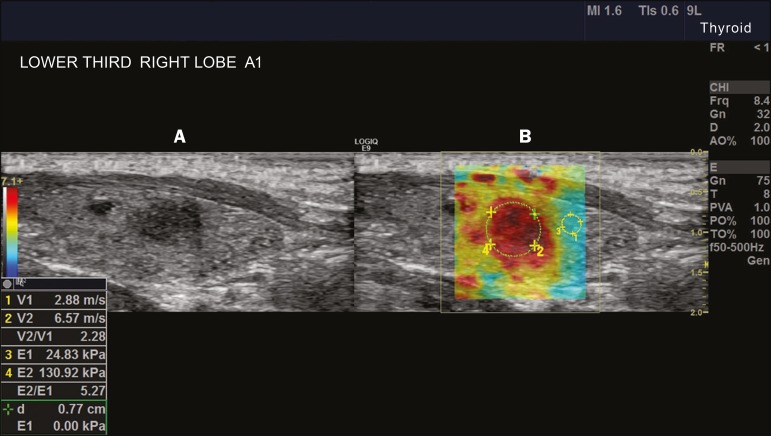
B-mode ultrasound (A) showing a hypoechoic thyroid
